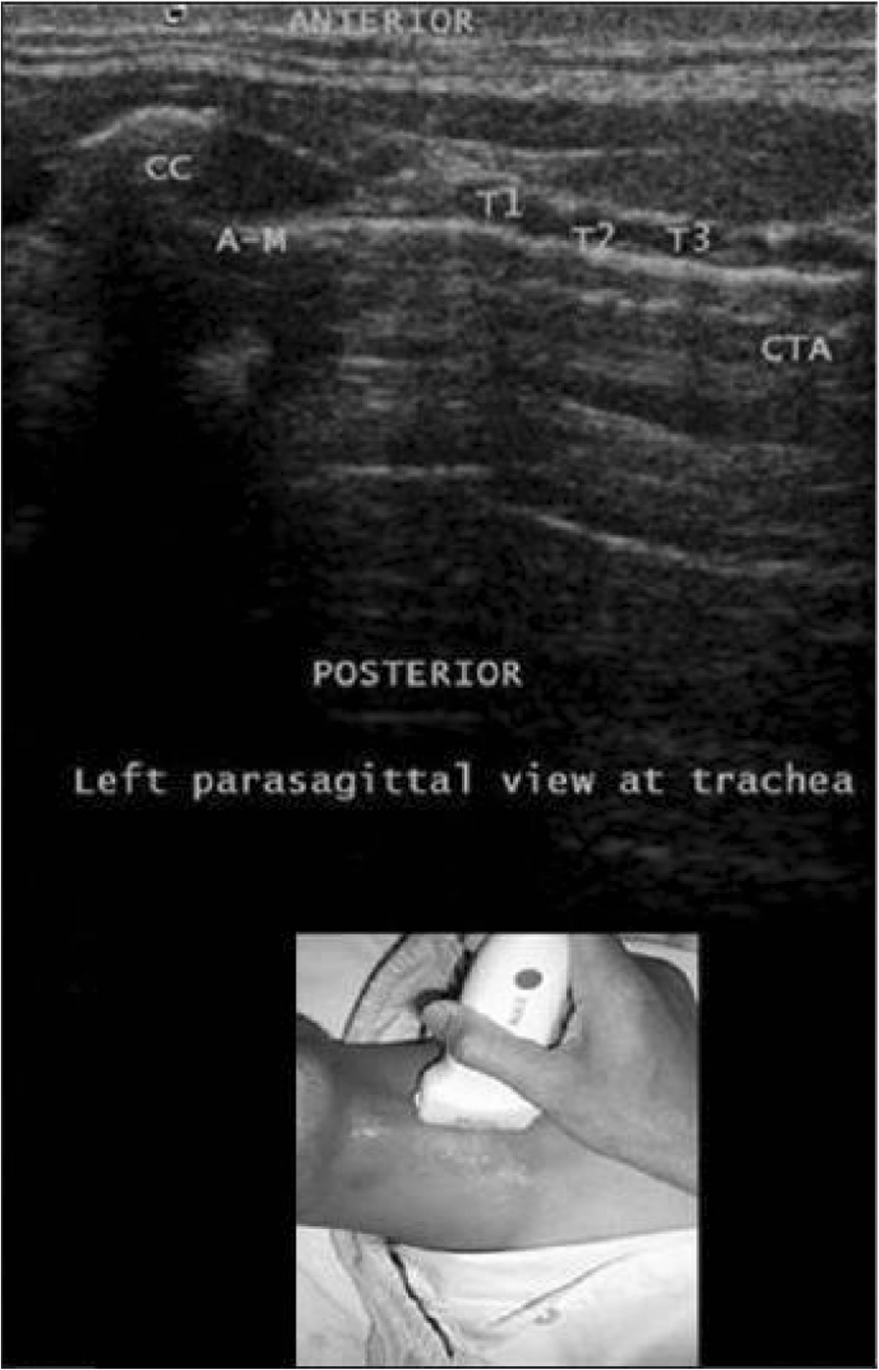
Left parasagittal view at the trachea. A-M, air-mucosal interface; CC, cricoid cartilage; CTA, comet tail artefact; T1-T3, tracheal cartilage. Reprinted with permission from Wolters Kluwer [42]
